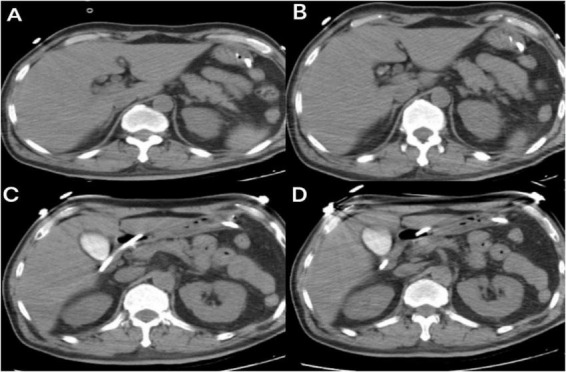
Non-contrast computed tomography of the pancreas in Case 2 reveals focal swelling of the pancreas (in the tail region), as shown in axial images (A–D).
